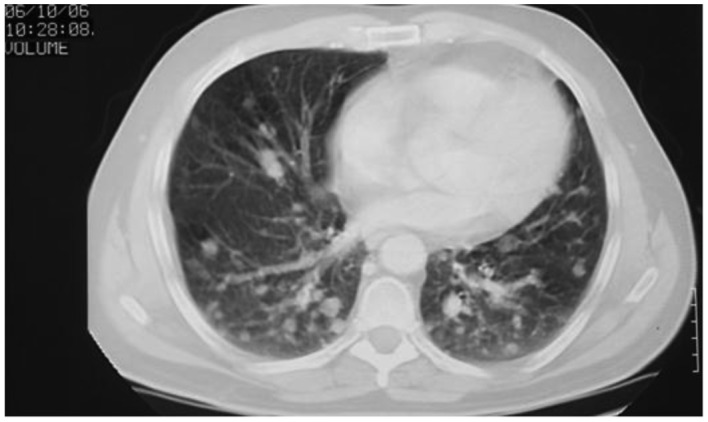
October 2006: The CT scan showed an increase in the number and size of bilateral multiple lung metastases.
